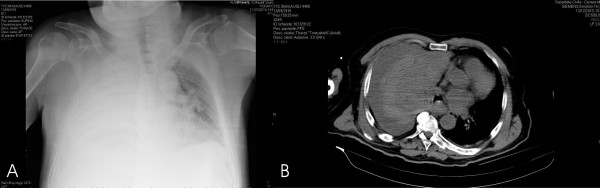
A-B. Preoperative chest X-rays and CT-scan showing massive right pleural effusion with complete atelectasis of the lung.
